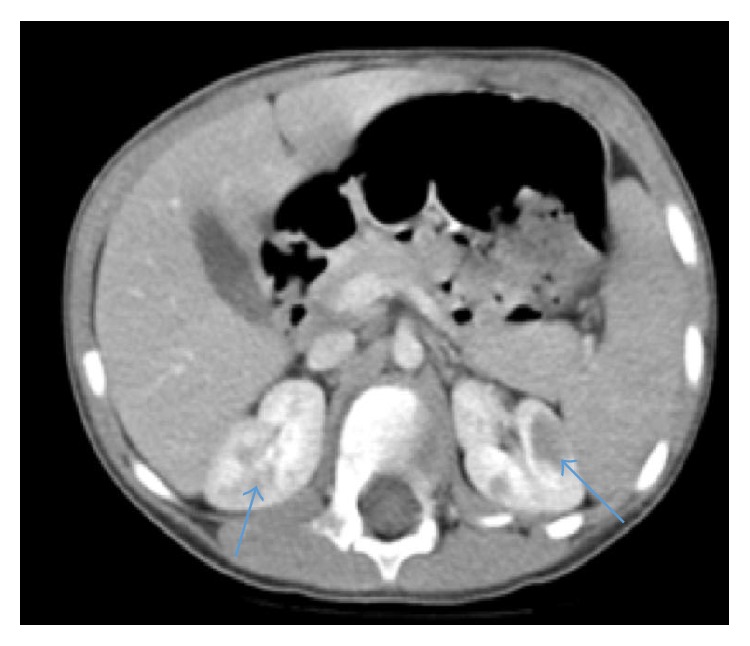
CT abdomen with contrast on initial presentation. Arrows showing bilateral hypodense lesions represent leukemic deposits.
